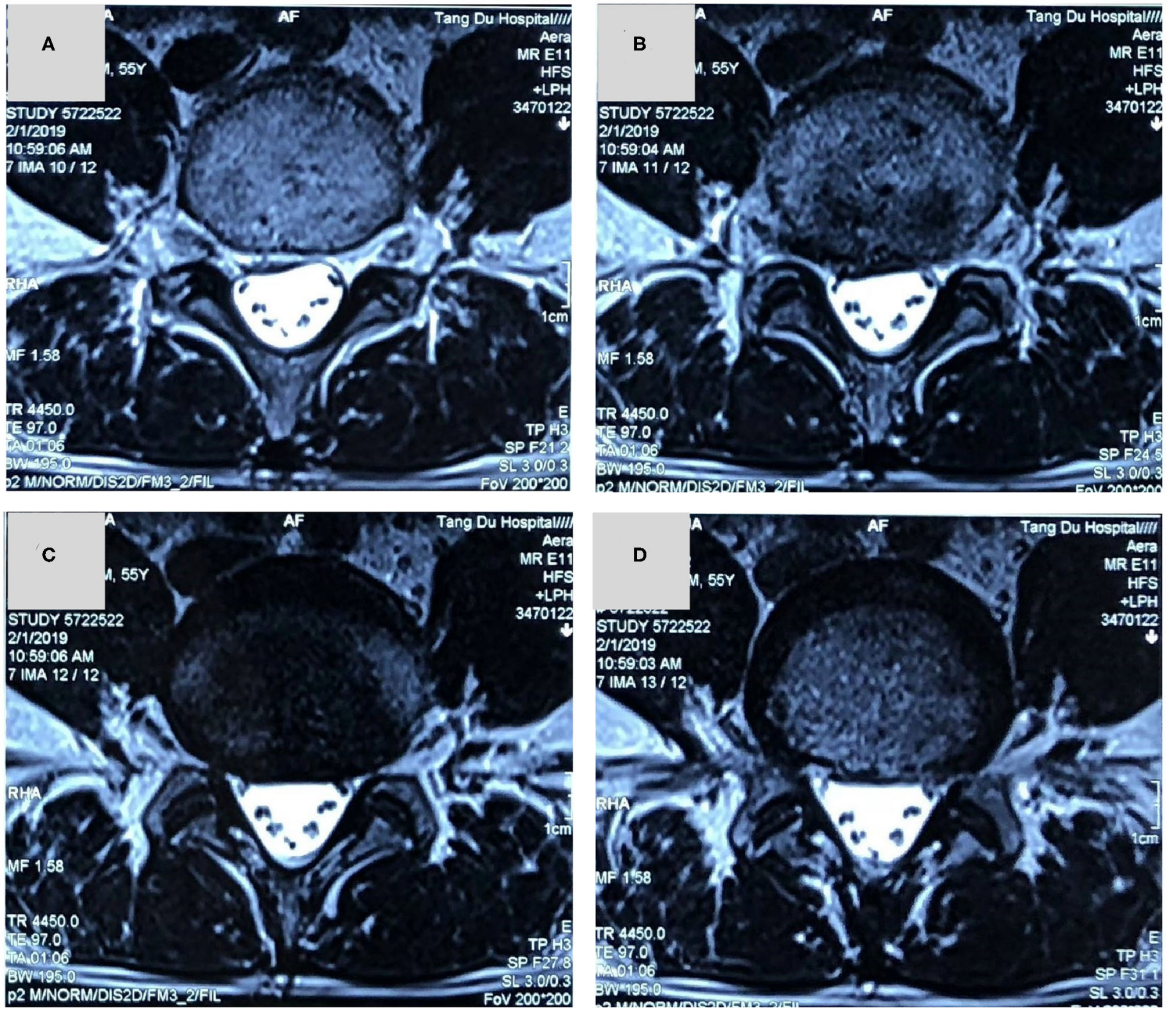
(A–D) A magnetic resonance imaging in axial slice showed that a lateral type disc protrusion on the right side in L4/5 and the right lumbar 5th nerve was mildly compressed.
